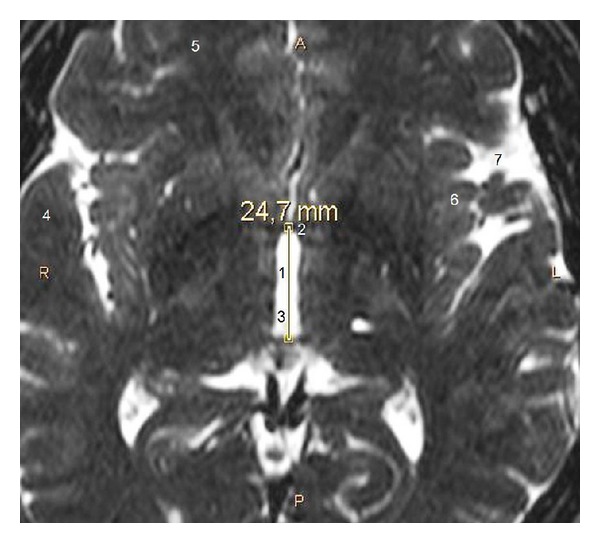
Transverse cerebral MRI section (T2-weighted, 1.5 Tesla) at level *Z* = 0, from a 51-year-old male, showing measurement of the AC-PC length. 1: AC-PC length, 2: AC, 3: third ventricle, 4: temporal lobe, 5: frontal lobe, 6: insula, 7: Sylvian fissure, A: anterior, P: posterior and, R: right, L left.
